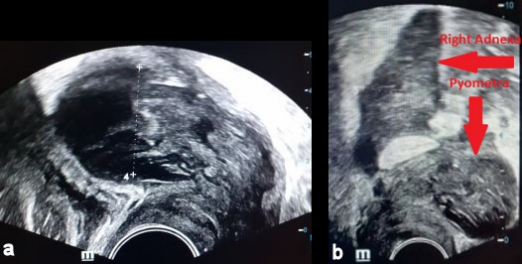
Transvaginal ultrasound (TVUS) a) of the uterus in the sagittal plane, b) of the right adnexa in the sagittal plane
